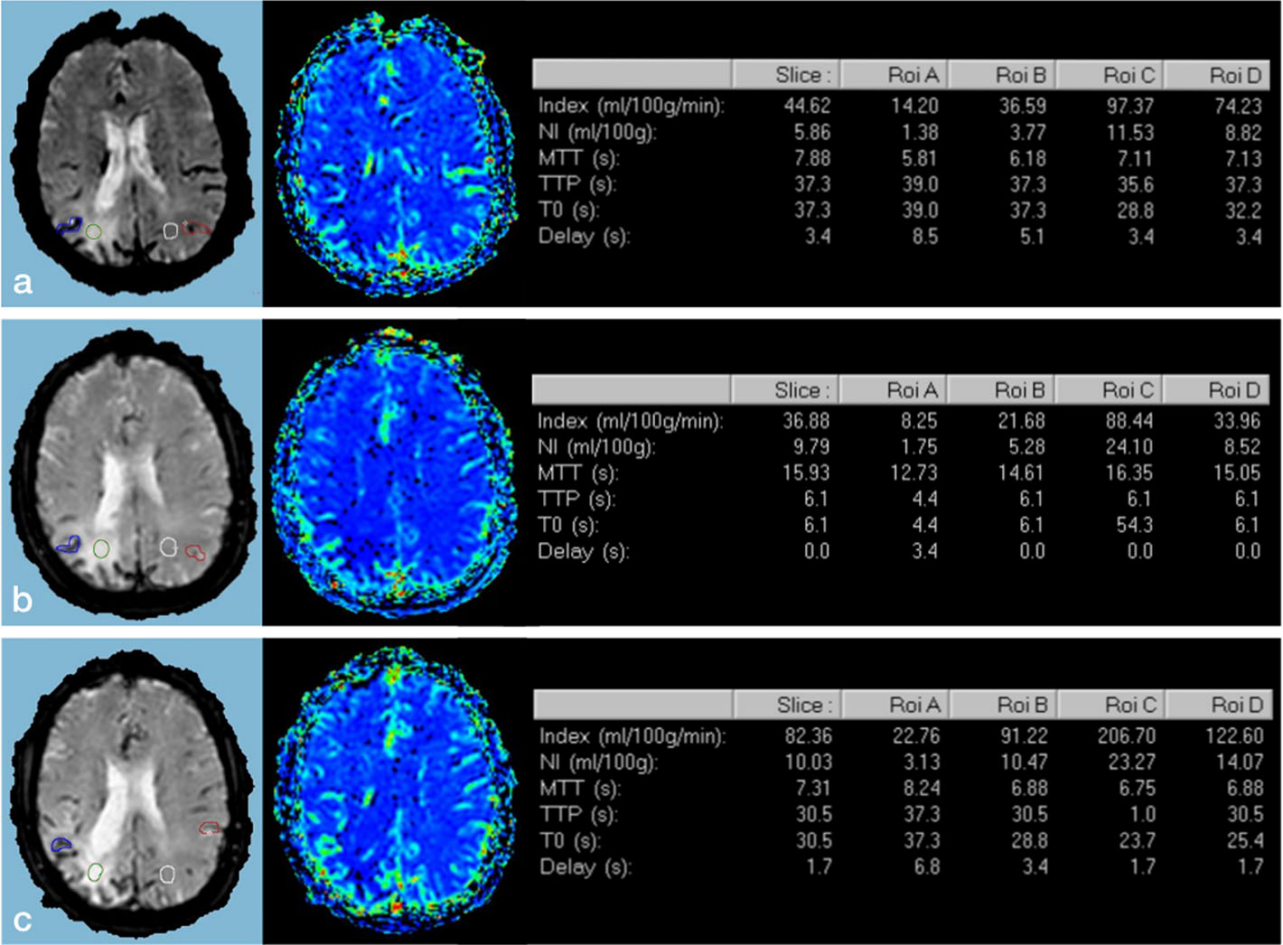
MRI perfusion maps and tables (Extended MR WorkSpace 2.6.3.5, Philips Medical Systems, Best, The Netherlands) at six months (a), one-year (b) and two years (c) follow-up. Notice reduced cerebral blood flow (CBF) and cerebral blood volume (CBV) in the right parietal cortical and subcortical white matter (green ROI) compared with contralateral normal hemisphere (white ROI) and increased leptomeningeal perfusion on the right side (blue ROI) compared to the left (red ROI)
